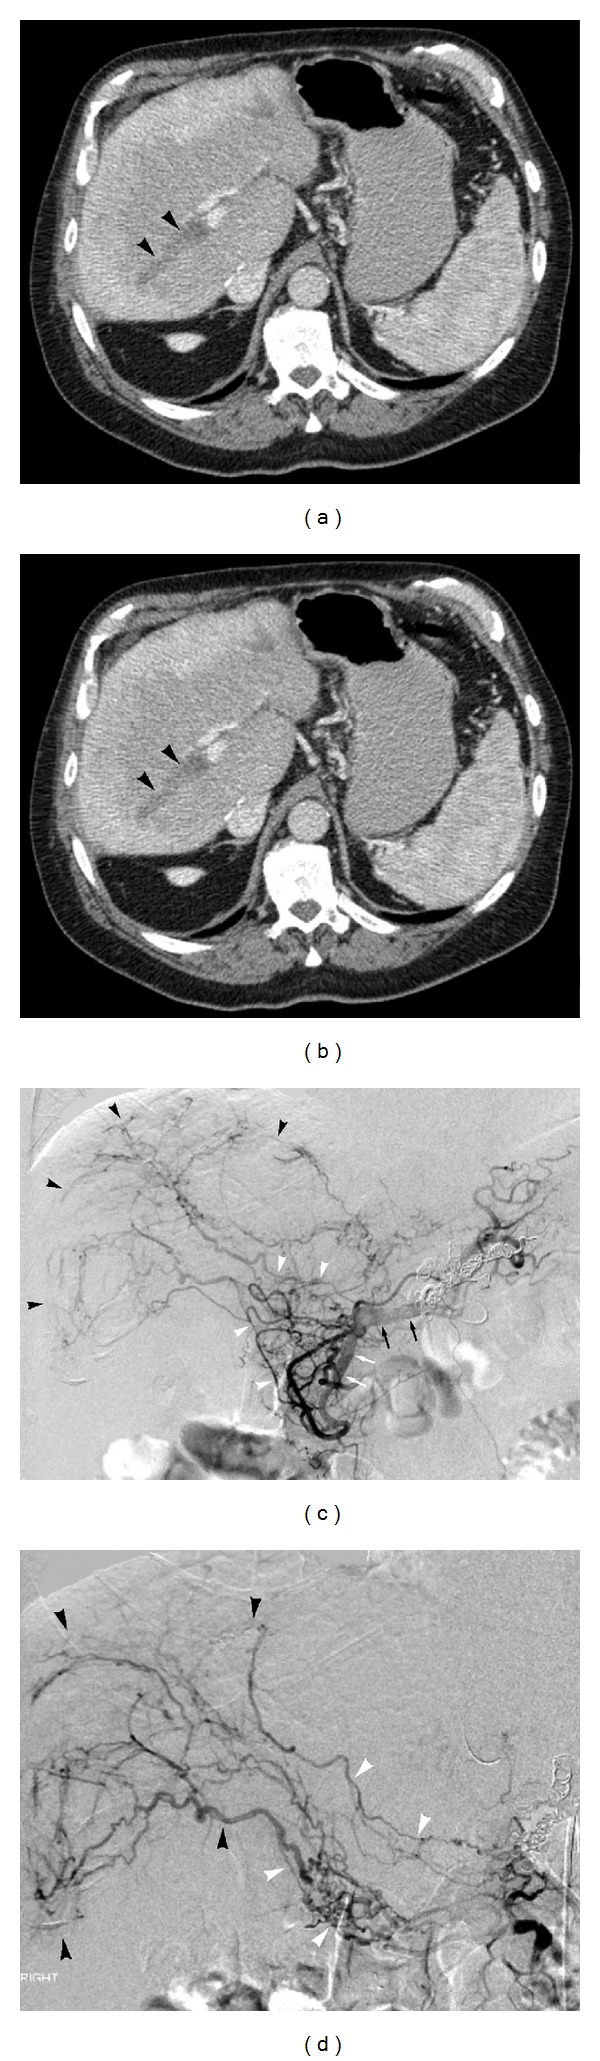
Axial contrast enhanced CT scan image (a) reveals right-sided intrahepatic portal vein thrombosis (arrowheads). Thrombus (arrowhead) extends into main portal vein on more caudal image (b). Subtracted common hepatic arteriogram (a) demonstrates chronic proper hepatic artery occlusion with transplant liver perfusion (black arrowheads) via mature pancreaticoduodenal collateral vessels (white arrowheads). Black and white arrows delineate common hepatic artery and gastroduodenal artery, respectively. Selective gastroduodenal arteriogram (b) better demonstrates mature pancreaticoduodenal collateral vessels (white arrowheads), which result in transplant liver perfusion (black arrowheads).
